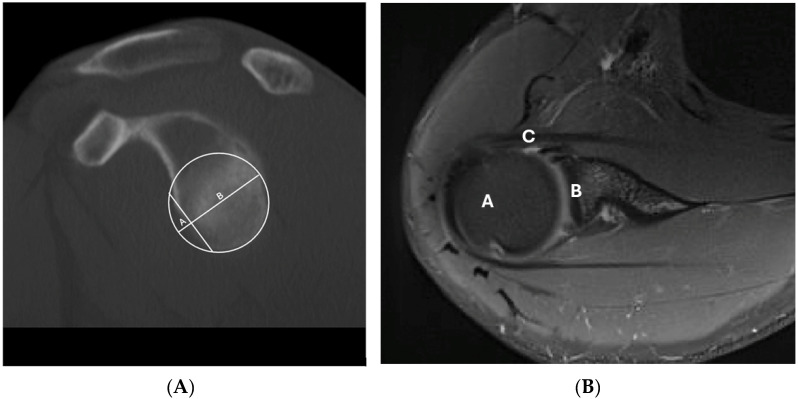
(A) Representative shoulder CT image demonstrating glenoid defect (sagittal bone); A—anterior bone defect of glenoid; B—glenoid bone. (B) Representative shoulder joint MRI image (pd_BLADE_fs_tra_256), A—humeral head, B—glenoid, C—labrum anterior lesion.
